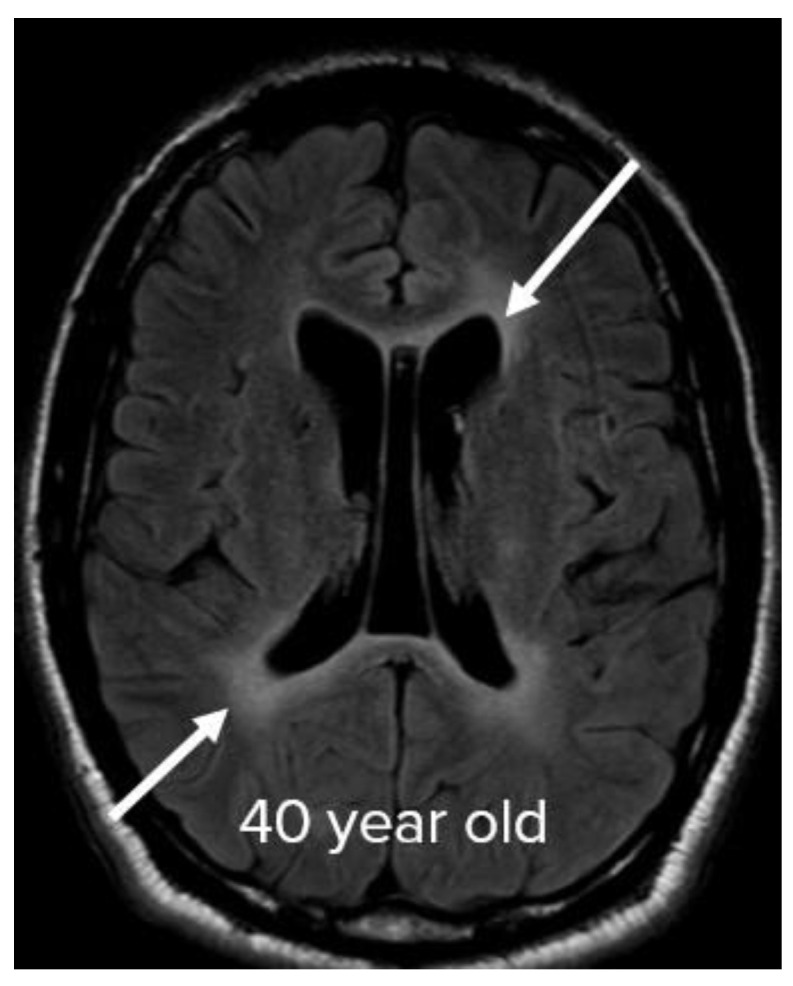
Periventricular involvement. Axial FLAIR image depicts periventricular FLAIR hyperintensities in a 40-year-old patient, otherwise not explained by chronic microangiopathic changes and absence of other risk factors. Image has been windowed manually to improve the visibility of signal abnormality.
